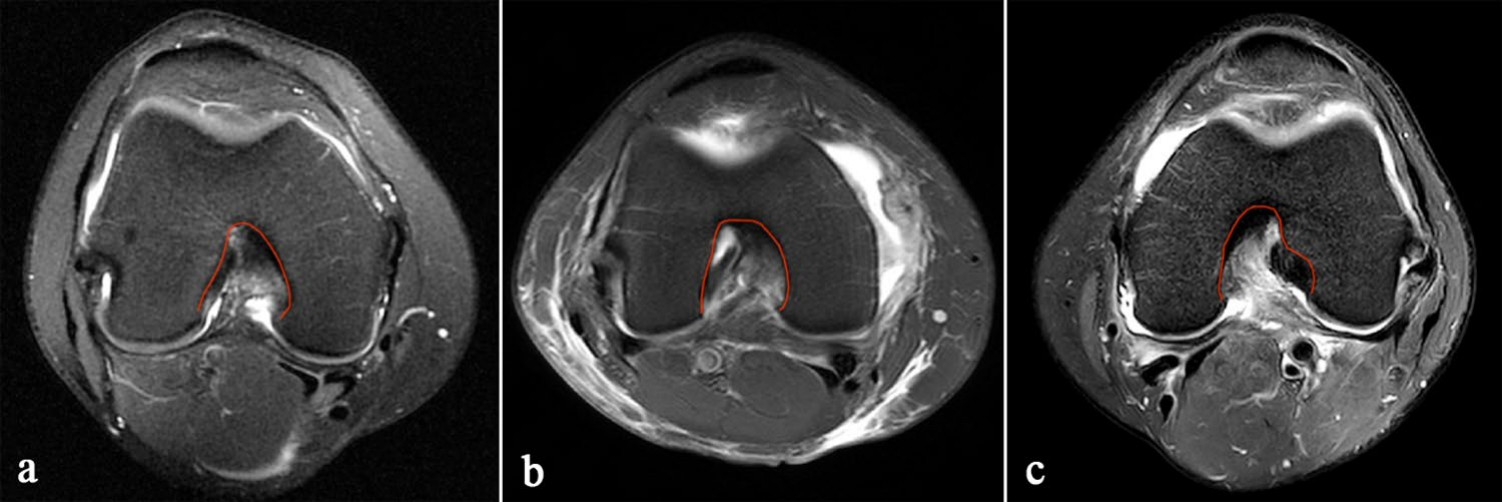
Axial MRI images of the knee, showing the three different intercondylar shape. a Type A. b Type U. c Type W
